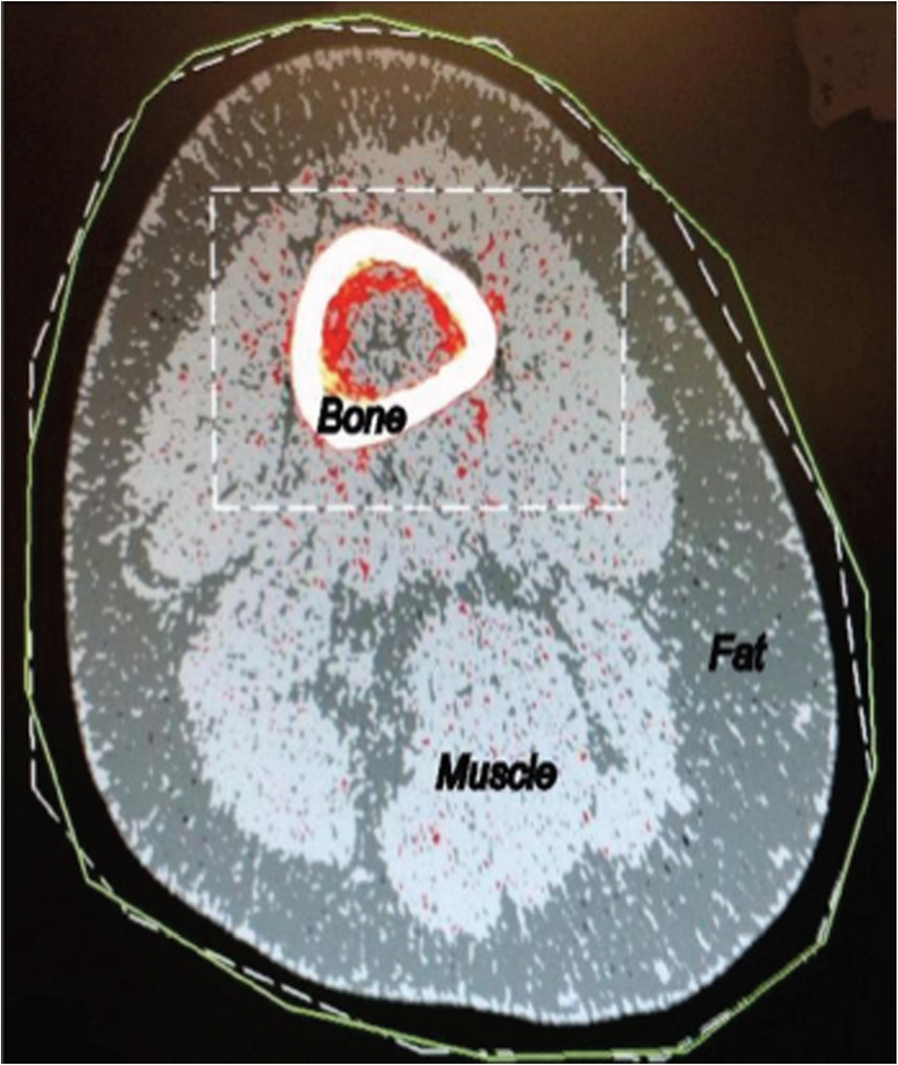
Representative peripheral quantitative computed tomography scan of the thigh for a participant with hip osteoarthritis depicting muscle and fat area
